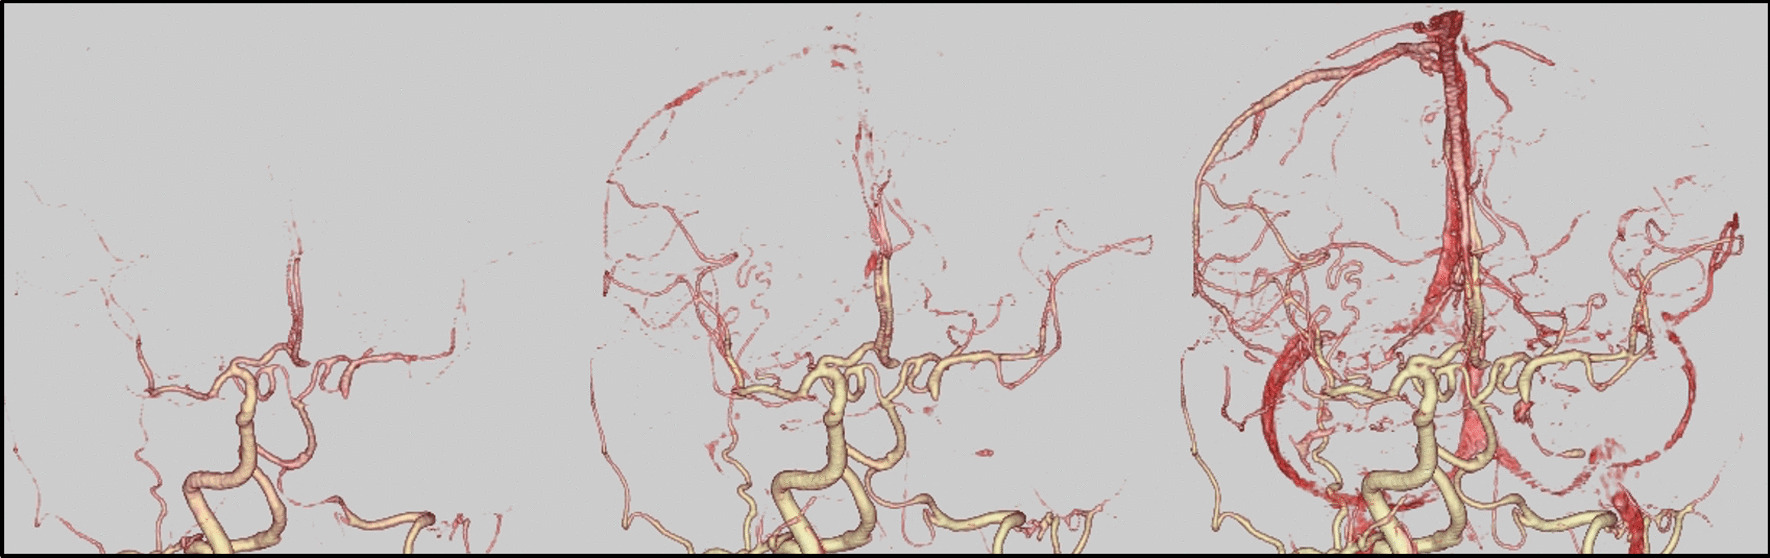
Four-dimensional computed tomography angiogram after left subclavian artery stenting. Collateral circulation is visible from the left posterior communicating artery to the left cerebral hemisphere. The left anterior cerebral artery (A1) is not visible
